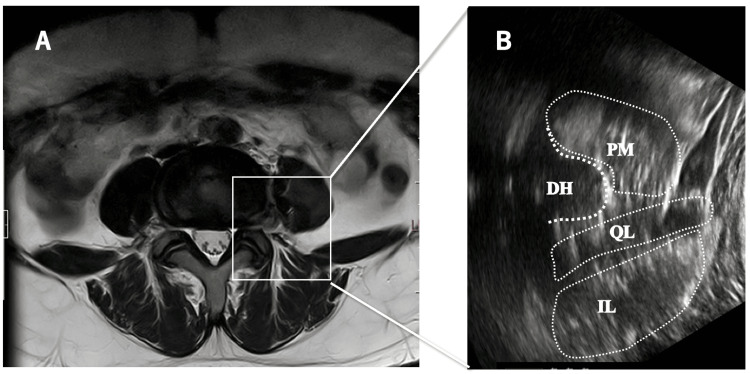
Magnetic resonance imaging (MRI) and short-axis ultrasonographic images. A: Axial MRI at the L4/5 level. B: Short-axis ultrasonographic image showing the psoas major muscle anterior to the herniated disc. PM, psoas major muscle; DH, disc herniation; QL, quadratus lumborum; IL, iliocostalis.
